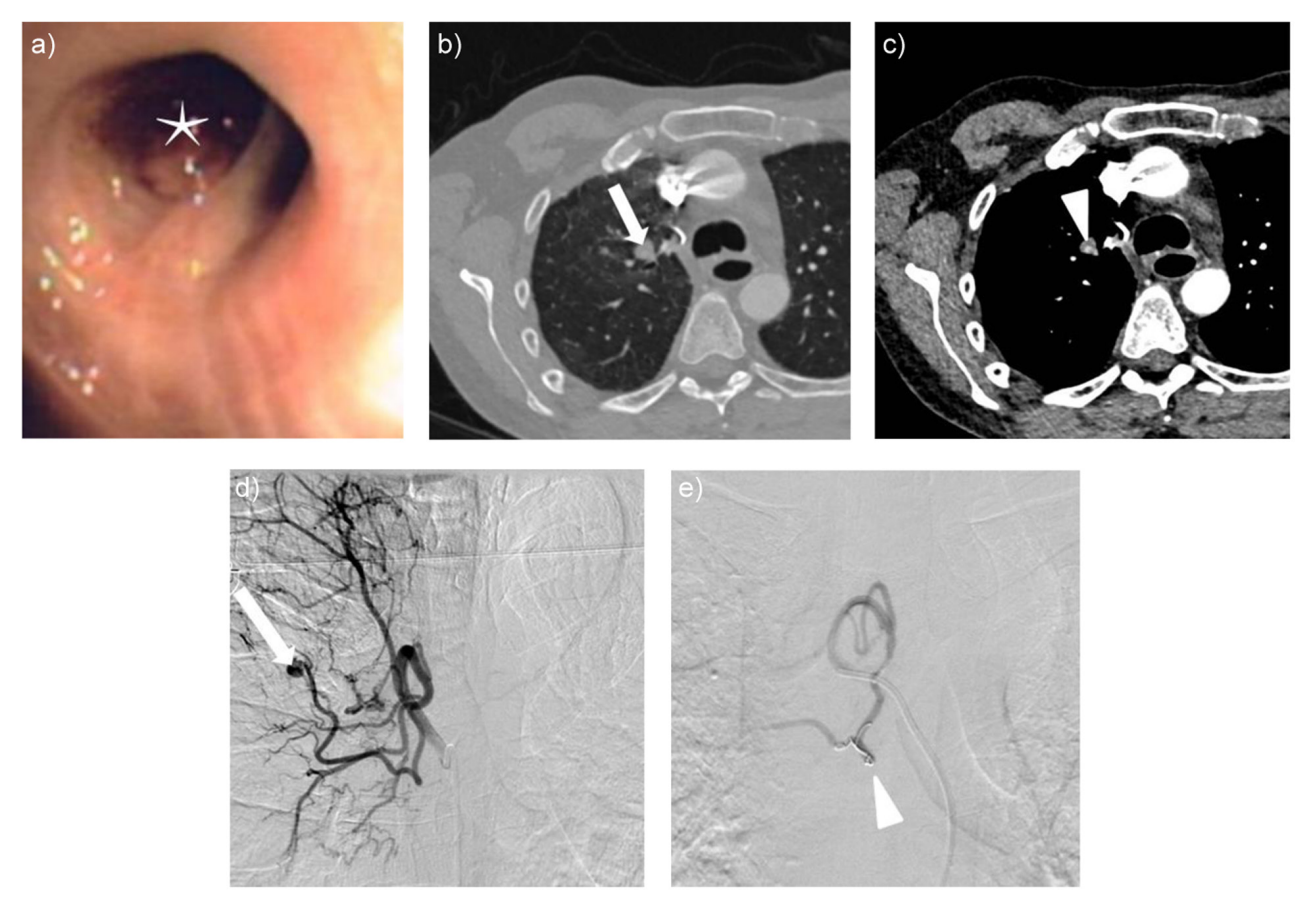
a) Bronchoscopy showed an organized blood clot obstructing a subsegmental bronchus of the right apical segment. b) and c) CT angiography showed bronchial obstruction at the level of the bifurcation of the apical and dorsal bronchial segments of the right upper lobe (b, arrow), along the corresponding arteries and containing a tiny (4 mm) contrast enhancement focus (c, arrow head), suggesting a BAA. d) and e) Digital subtraction angiography after artery catheterism showed the BAA in the right upper lobe (d, arrow). Selective embolisation was performed with microcoils (e, arrow head).
